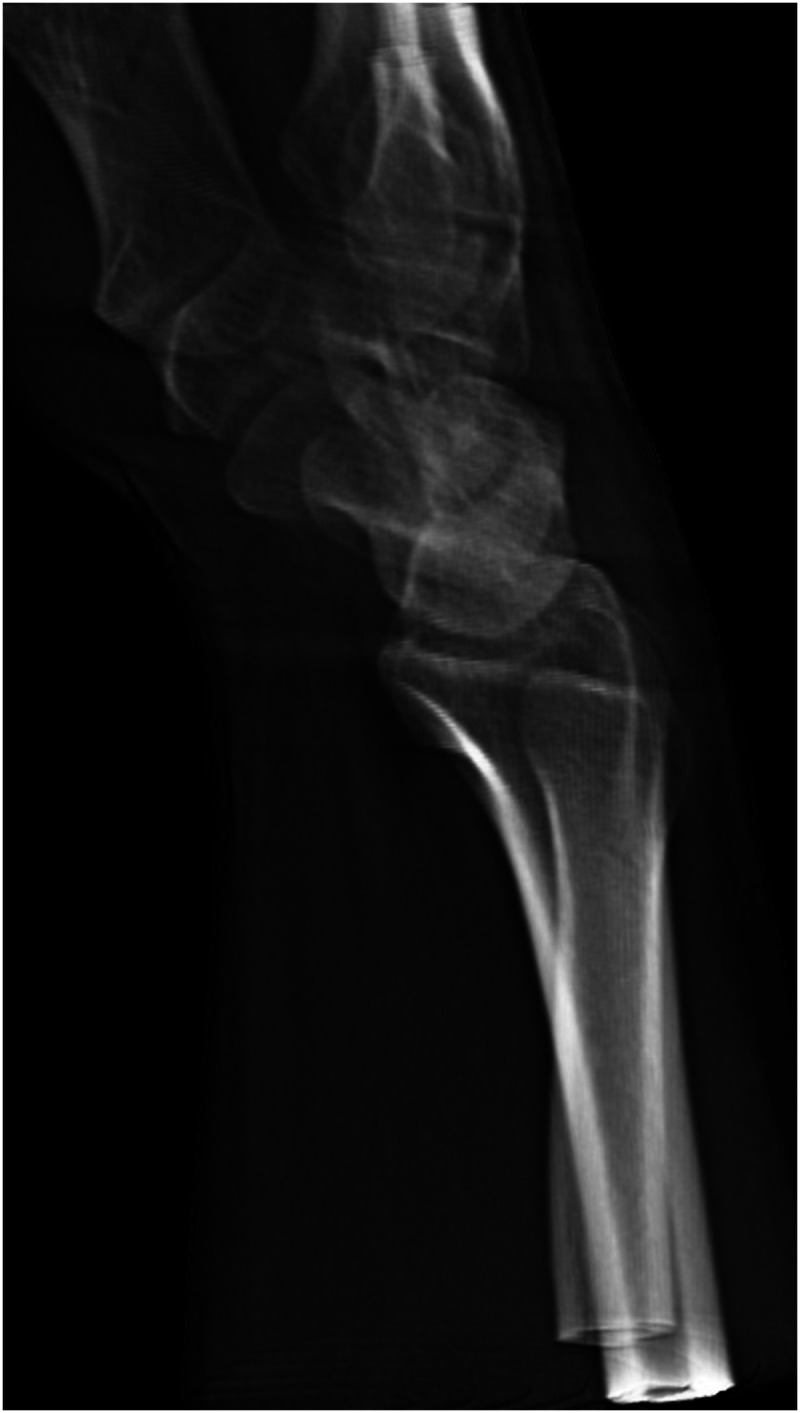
A summation radiograph reconstructed from cone-beam CT data.
